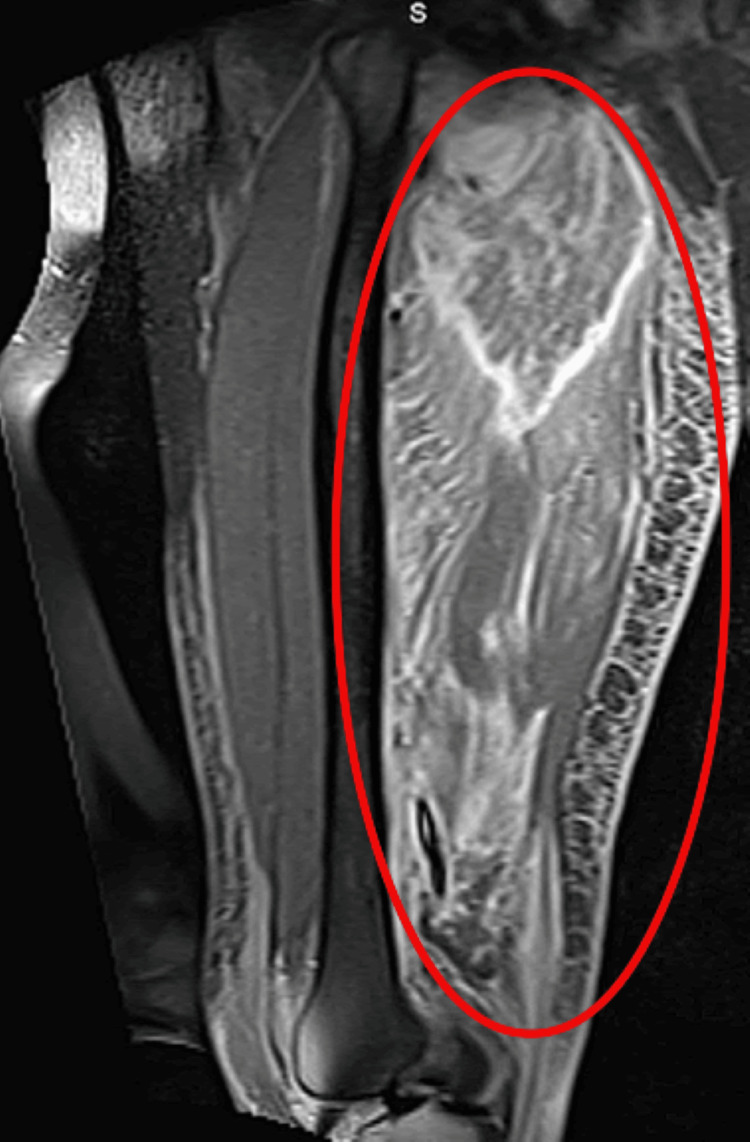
Sagittal T2-weighted MRI of the right femur demonstrating muscle edema secondary to pyomyositis. The hyperintense signal (red circle) on sagittal T2-weighted MRI of the right femur demonstrates diffuse muscle edema and inflammatory changes, suggestive of pyomyositis involving the hamstring, proximal quadriceps, and adductor muscle compartments. MRI: magnetic resonance imaging.
